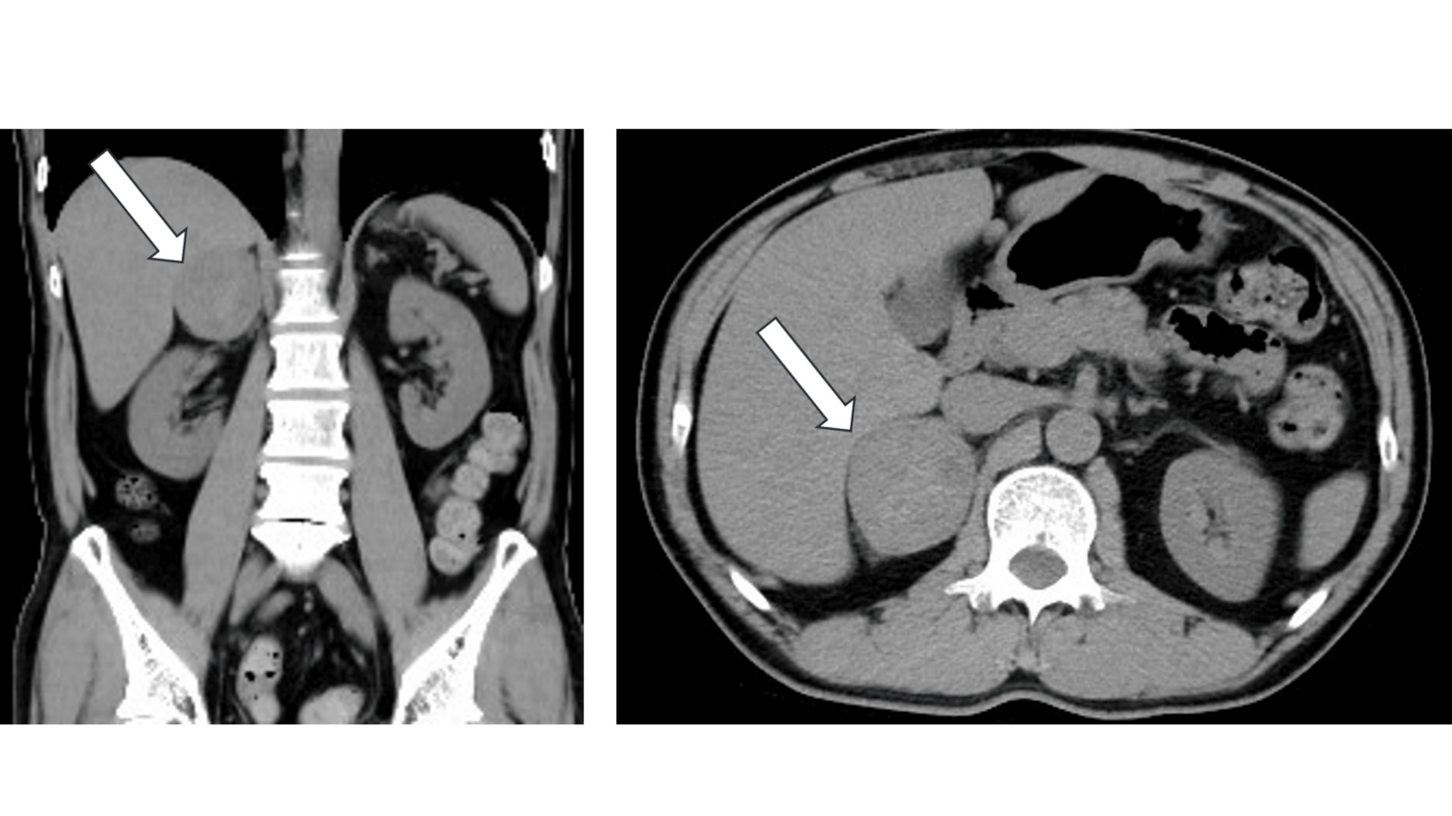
Simple computed tomography (CT) scan of the abdomen. The CT scan reveals a well-defined, borderline, slightly heterogeneous mass lesion measuring approximately 5 cm in diameter in the right adrenal gland. This finding, combined with elevated urinary metanephrine and normetanephrine, is consistent with the diagnosis of pheochromocytoma.
